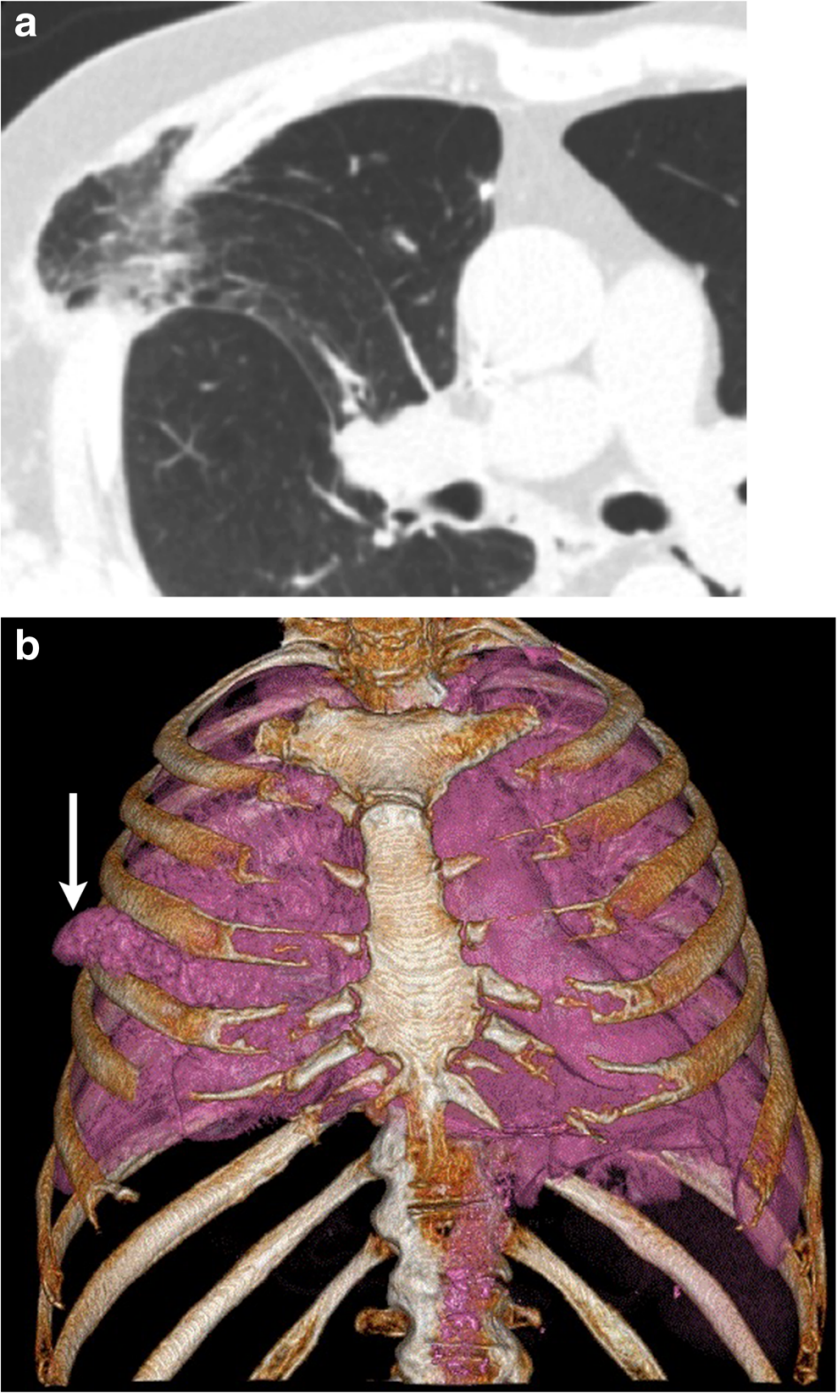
a, b A 70-year-old female with a history of intercostal chest tube drainage for pleural effusion. Axial CT (a) and volume-rendered image (b) in a patient with previous right upper lobe wedge resection for a stage I lung cancer identifies focal intercostal herniation of the right upper lobe (white arrow). The neck of the hernia is narrow, indicating incarceration. In addition, there is abnormal orientation of the bronchovascular pedicle with narrowing of the bronchiole. Ground-glass opacities in the herniated lung indicate atelectasis (Movie 1). Elective repair is recommended for these hernias unless asymptomatic
